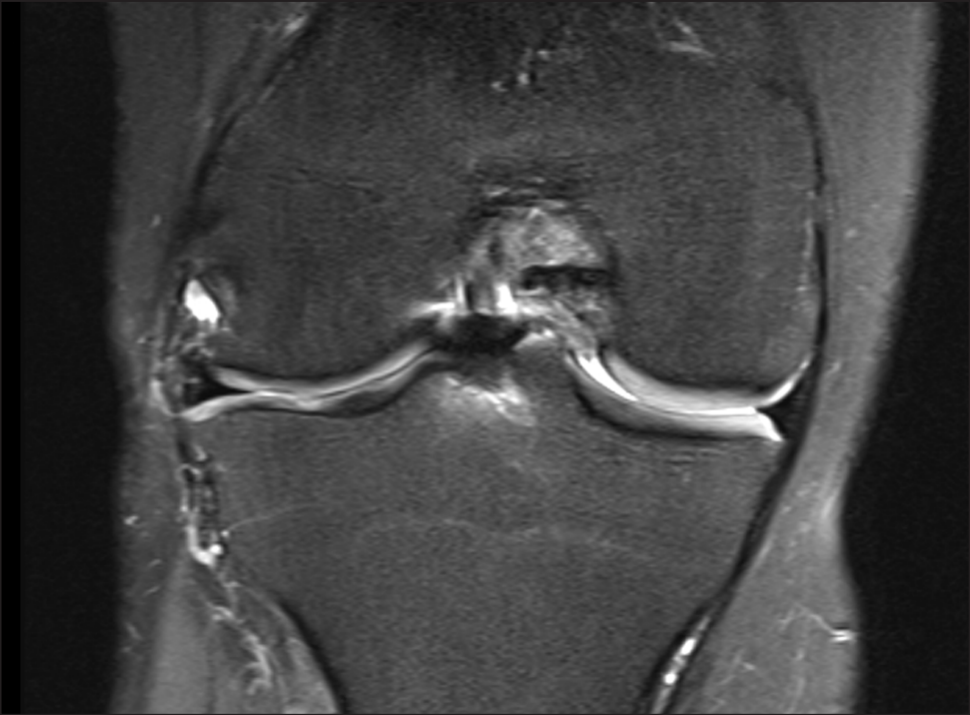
Coronal fat saturation T2-weighted fast spin echo MR-image of a meniscus allograft in the lateral compartment. Radial displacement of the allograft is a quite common finding after meniscal allograft transplantation.
